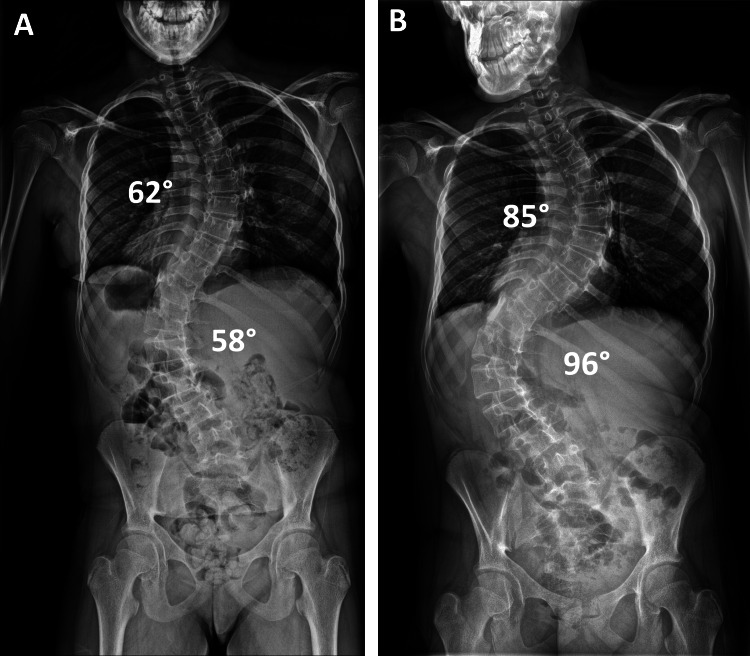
Radiographs showing the rapid progression of scoliosis. Posteroanterior radiographs of the spine taken at initial diagnosis in the patient's local service (A) and when she attended our clinic for the first time (B) show a very significant progression of her thoracic and lumbar scoliosis, which occurred over a period of rapid spinal growth within only eight months.
